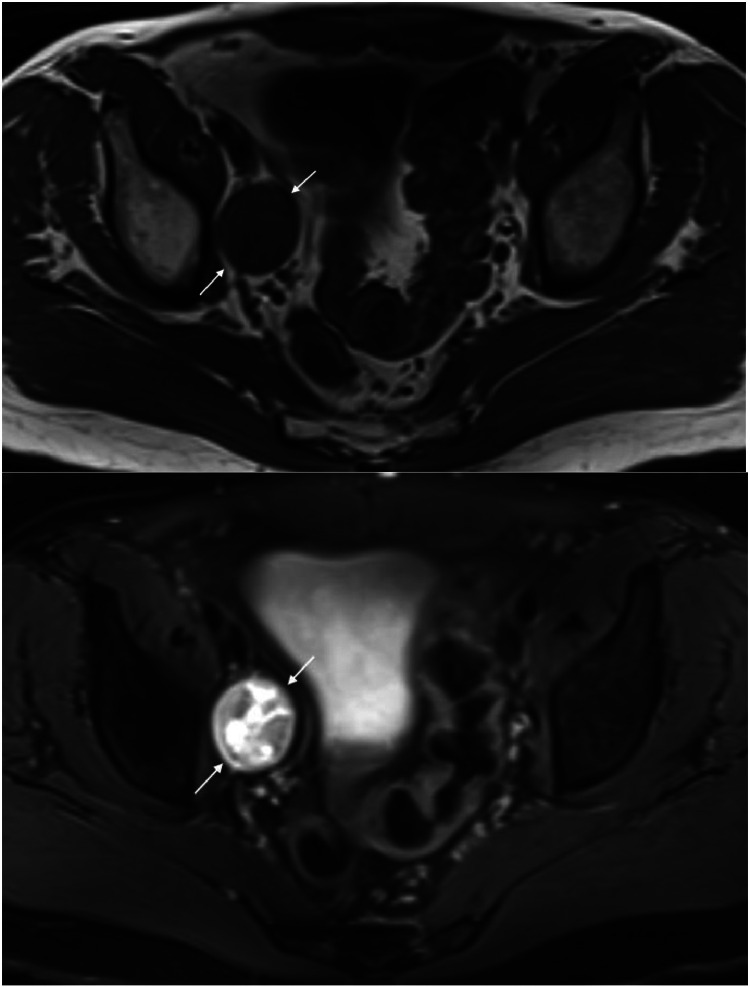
Axial T1-weighted (A) and T2-weighted fat suppressed (B) images demonstrate an ovoid well circumscribed 6.4 × 3.3 × 3.1 cm right pelvic mass with intermediate to hypointense T1 signal, and heterogeneous T2 signal (white arrows).
